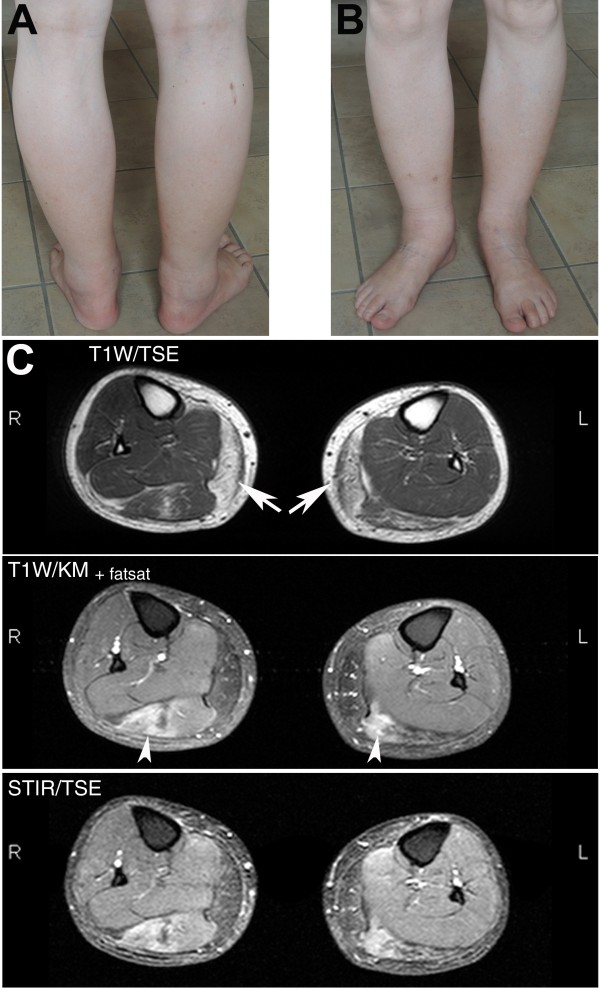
Photographs and magnetic resonance imaging scans of the patient’s legs. (A,B) Photographs taken of patient’s legs. Note muscle wasting that predominantly affects posterior calf muscles (A). (C) Magnetic resonance images of both calves (top: T1-weighted image; middle: T1-weighted image, contrast enhanced, fat suppressed; bottom: Short T1 inversion recovery (STIR) images). Fatty degeneration of the medial heads of the gastrocnemius muscles (arrows). Additional areas of edema and contrast enhancement in the lateral head of the gastrocnemius muscle indicate muscle inflammation (arrowhead).
